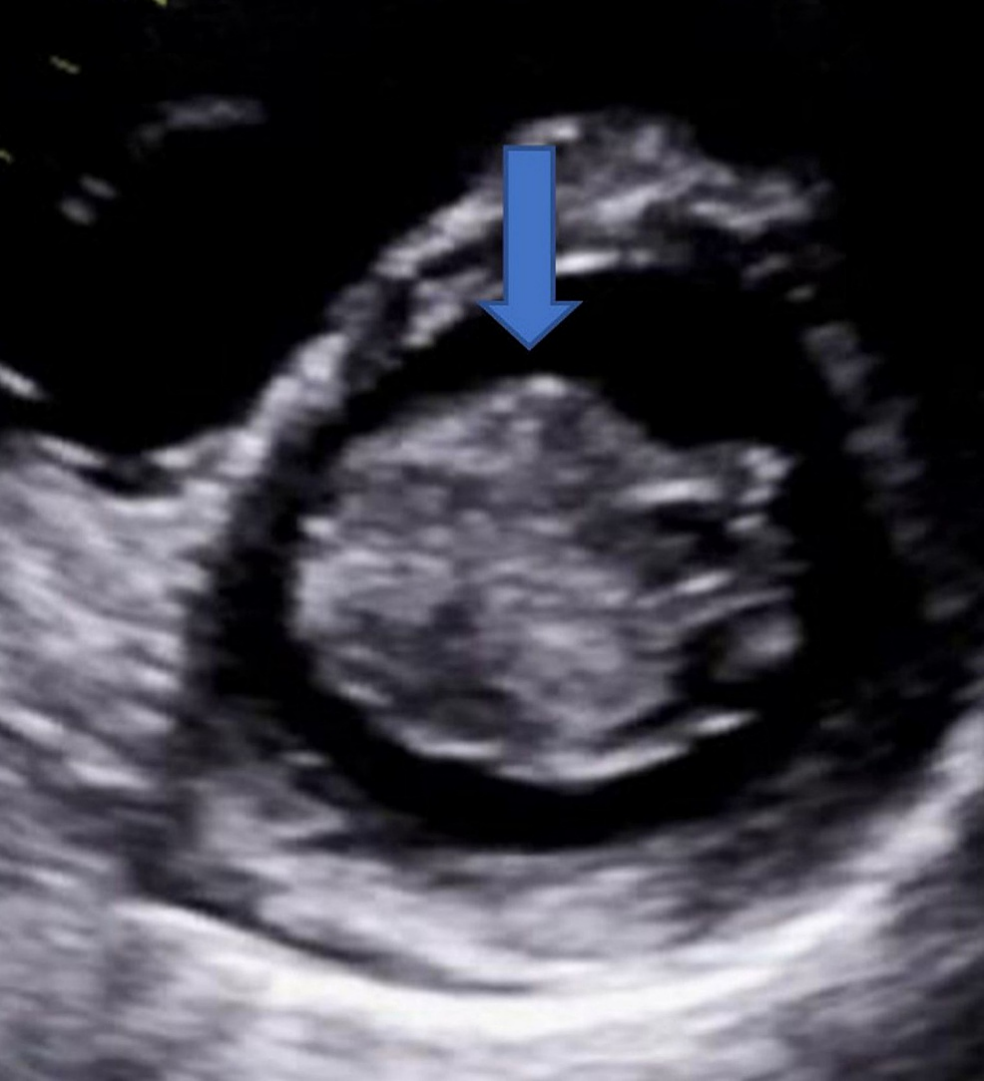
Echocardiography parasternal short-axis view showing left atrial myxoma
